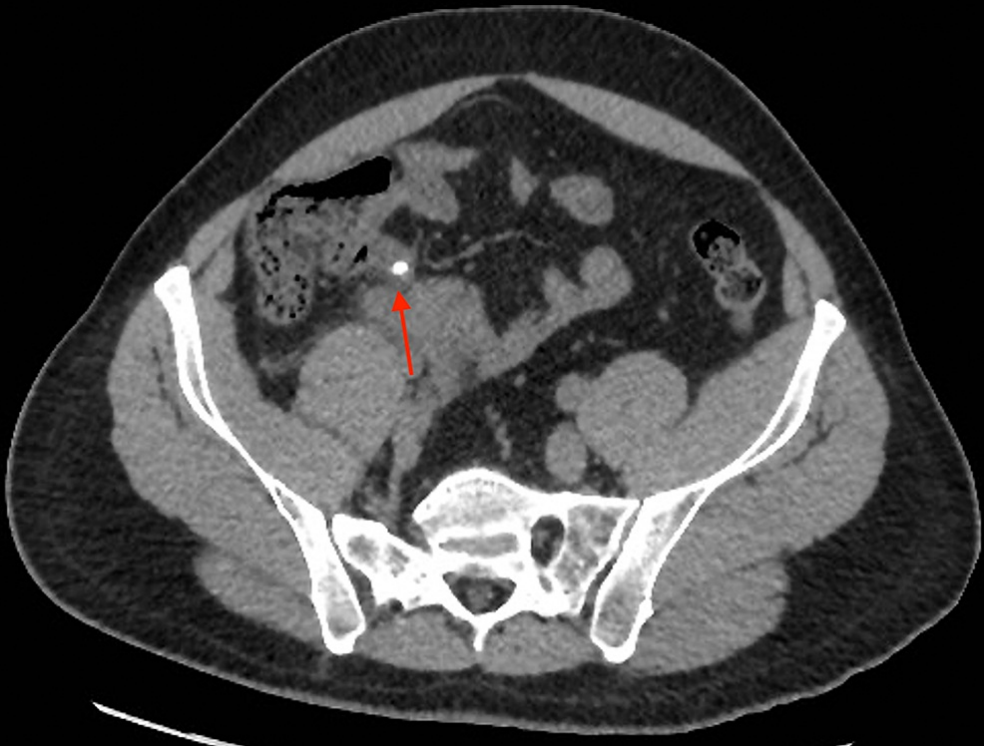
Axial CT imaging of the lower abdomen without contrast Axial cut image of the lower abdomen demonstrates the presence of a 1 cm stone with a protruding cecal pouch, likely representing an appendices stump. There is minimal wall thickening of the cecal wall.
